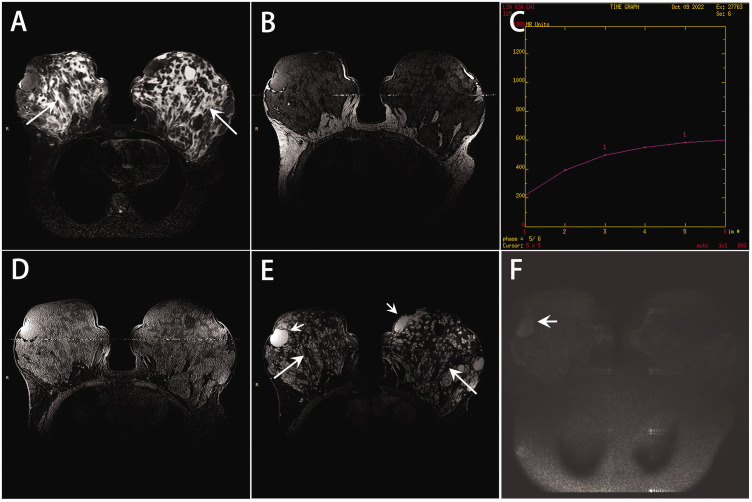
Magnetic resonance imaging findings of pseudoangiomatous stromal hyperplasia. (a) T2-weighted imaging showed high-signal slit-like spaces (long arrows). (b) T1-weighted imaging showed low-signal areas, and (d) T1-weighted fat suppression imaging also demonstrated low signals. (e) These slit-like spaces (long arrows) showed no enhancement, while multiple oval-shaped masses (short arrows) with clear boundaries were observed. (c) The dynamic enhancement curve was ascending. (f) Diffusion-weighted imaging revealed mild diffusion restriction within the enhanced masses.
